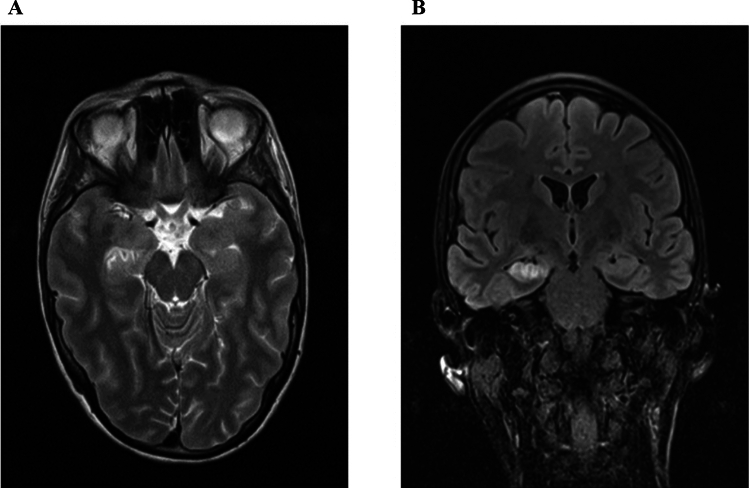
Limbic MRI pathologies in a patient with GAD antibodies, Representative MRI findings in a patient with right-hippocampal necrosis and temporomesial space-occupying effects in the right amygdala complex, hippocampus, and nucleus accumbens. A T2-weighted sequence, axial; B FLAIR-sequence, coronary (source: University Hospital Dresden, Department of Neuroradiology)
